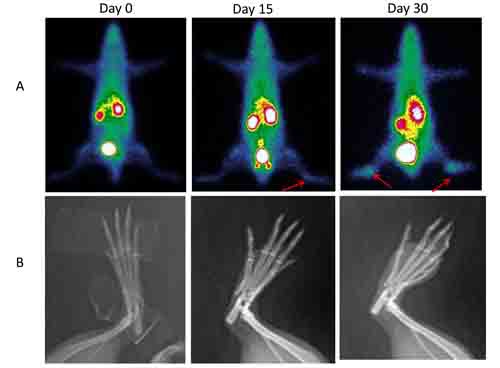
Temporal changes in rheumatoid arthritis monitored by serial ^99m^Tc-3PRGD2 and X-ray imaging A. ^99m^Tc-3PRGD2 scintigraphy prior to, and 15 and 30 days after immunization Arrow indicates increased joint uptake. B. Matched X-ray images.
